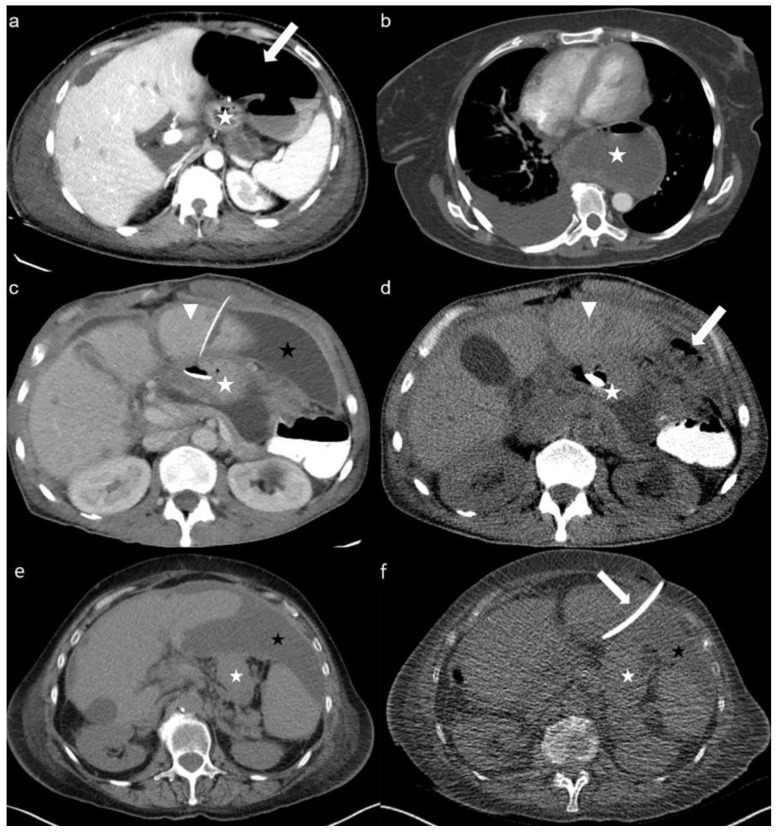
Sequential axial enhanced (a–c) and unenhanced (d) CT images demonstrate insufficient punction fields yielding unsuccessful CT-PG insertions in 4 patients: (a) Colonic interposition *(arrow)* ventral to the stomach *(star)*; (b) Hiatus hernia *(star)*; (c,d) the endoscopically inserted PEG tube is located in the gastric lumen *(white star)* and passes through the liver (*triangle*) as a complication of the previous endoscopic insertion. After paracentesis, insufficient punction field due to hepatomegaly *(triangle)* and colonic interposition *(arrow)* in the same patient; (e,f) presence of excessive haemorrhagic ascites *(black star)* despite multiple paracentesis and drainage *(arrow)*.
